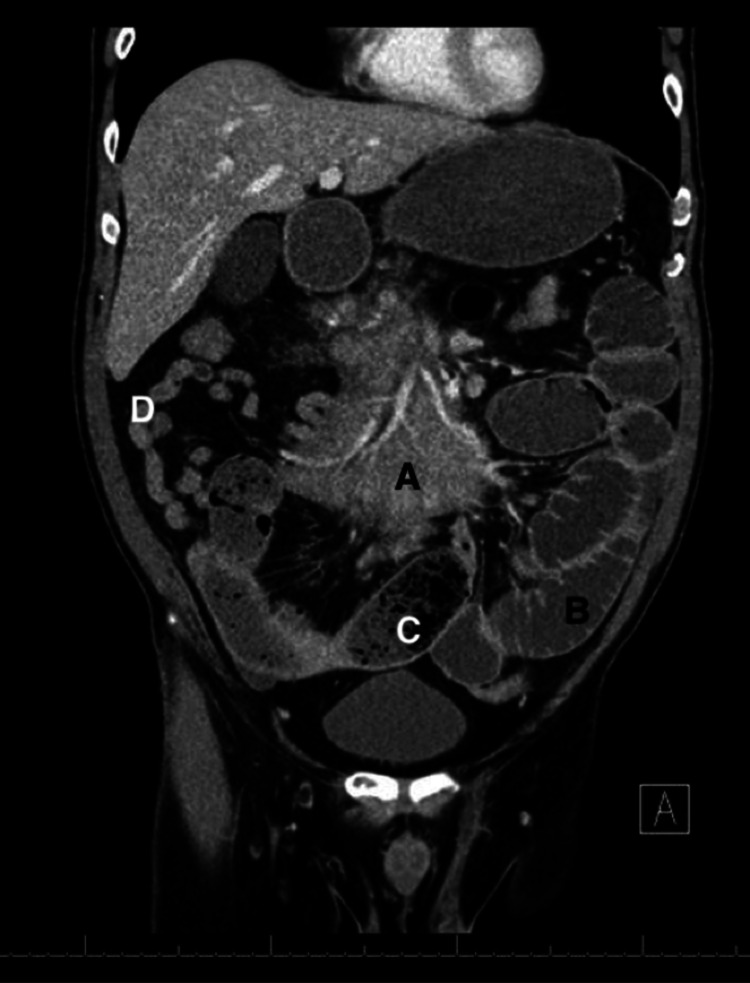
The coronal image of the contrast-enhanced computed tomography scan of the abdomen and pelvis. (A) Thickening of the small bowel mesentery consistent with a mass. (B) Dilated loops of the bowel with air-fluid levels. (C) Fecalization of the small bowel contents. (D) Collapsed loops of the distal small bowel.
